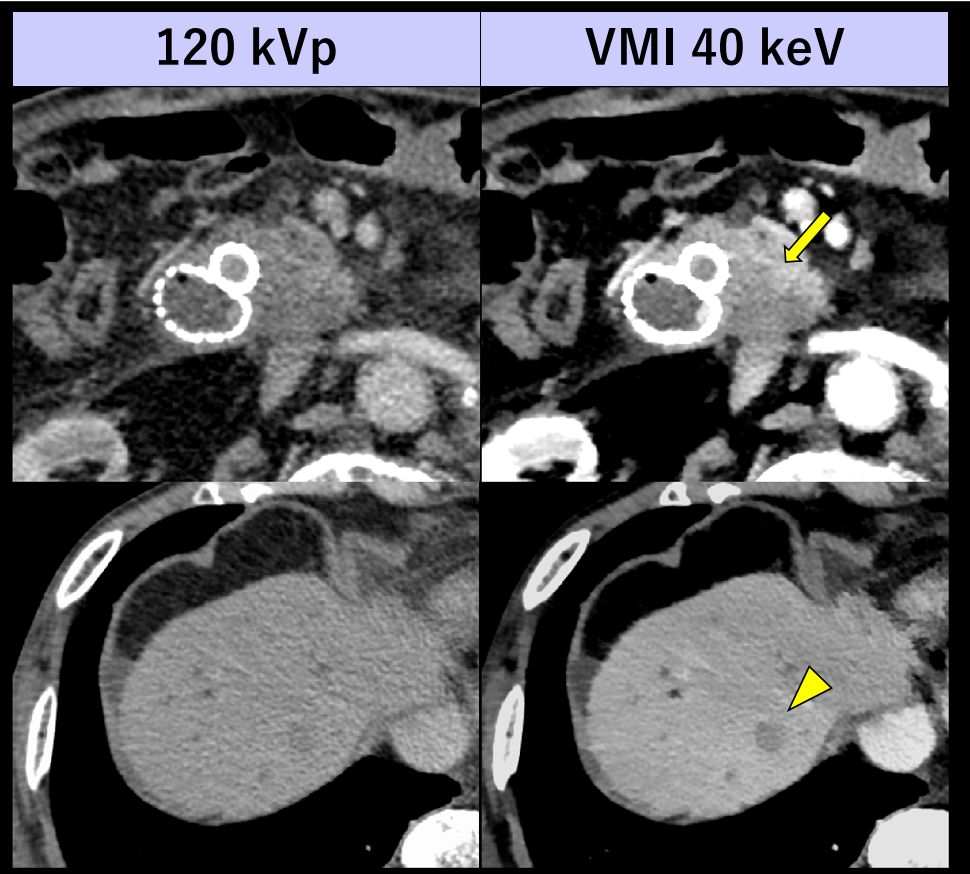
An 83-year-old man with pancreatic ductal adenocarcinoma in the pancreatic head and hepatic metastasis underwent multiphase pancreas CT (upper row: pancreatic phase; lower row: portal venous phase) using 33 mL of ICM (200 mgI/kg) on dual-layer detector scanner. In the 40 keV VMI, both the pancreatic and hepatic lesions are clearly delineated, in contrast to the conventional 120 kVp images where both lesions are barely visible due to insufficient contrast enhancement
